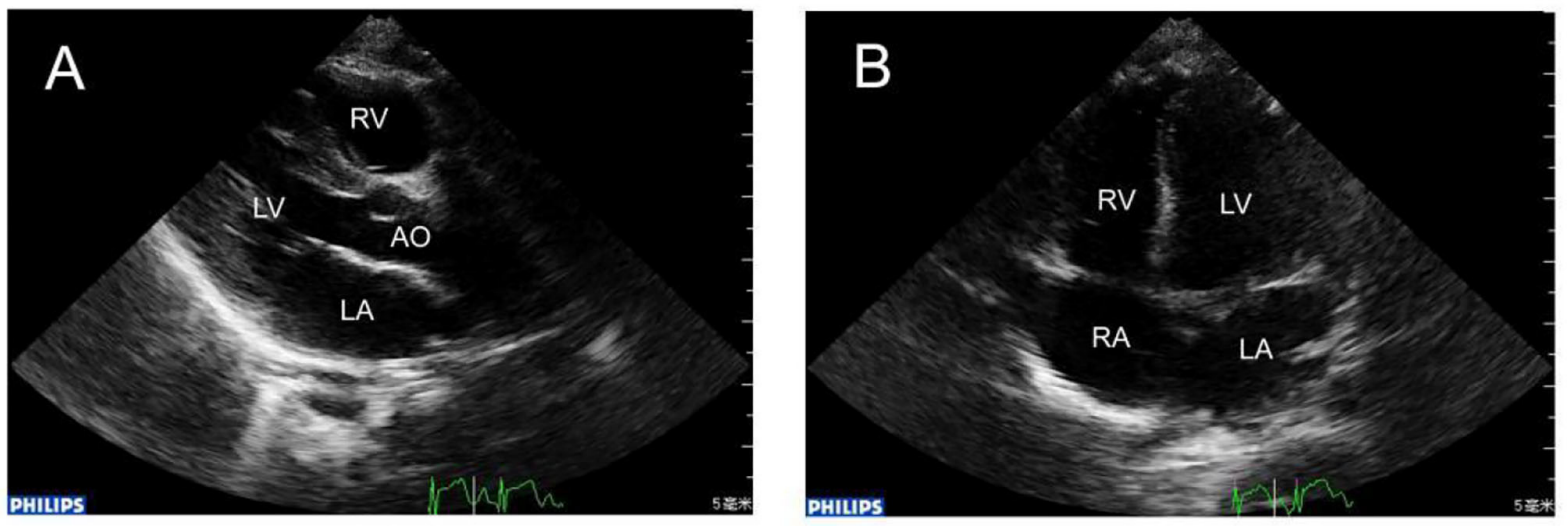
(A) 1 year later, repeat echocardiography showing that the RCA was in normal size. (B) The apex four-chamber view showed that in the same position the huge thrombus disappeared.
